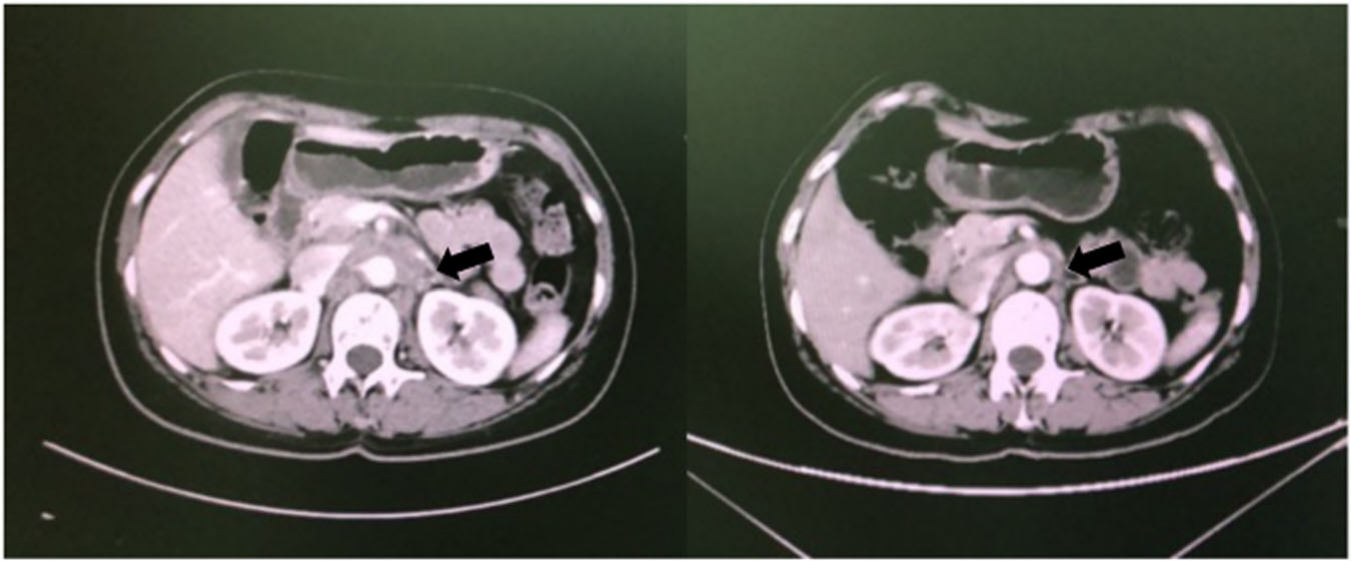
The efficacy of LZM005 in a phase Ia patient. Significant target lesion response (PR) in a patient with LZM005 20 mg/kg in CT scan (black arrow indicated the lesion, left: pre-treated, right: post-treated).
